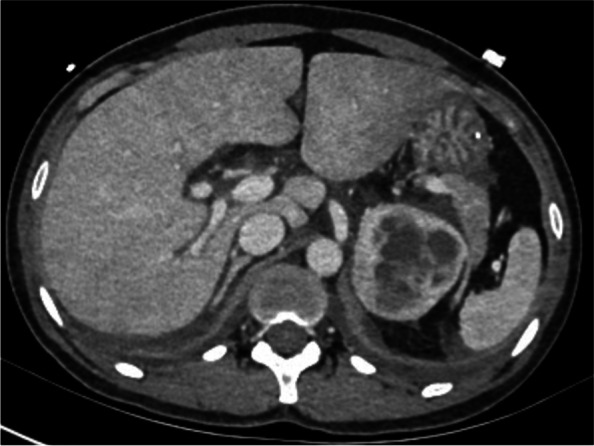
A left-sided adrenal mass of 6.5cm × 5.9cm complicated with tumor stroke was seen on the CT scan
